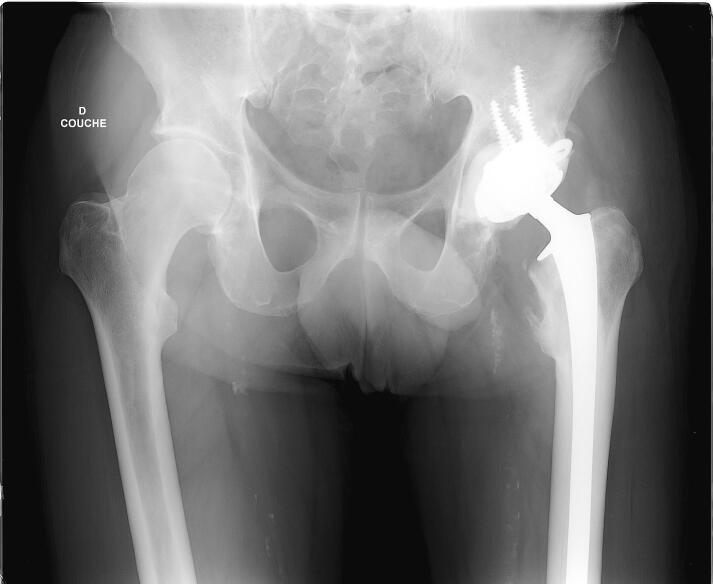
A: pre-operative radiograph of a 61-year-old man with lung cancer involving metastasis in the acetabulum. B: Post-operative radiograph. The patient underwent total hip arthroplasty with a Müller reinforcement ring.
